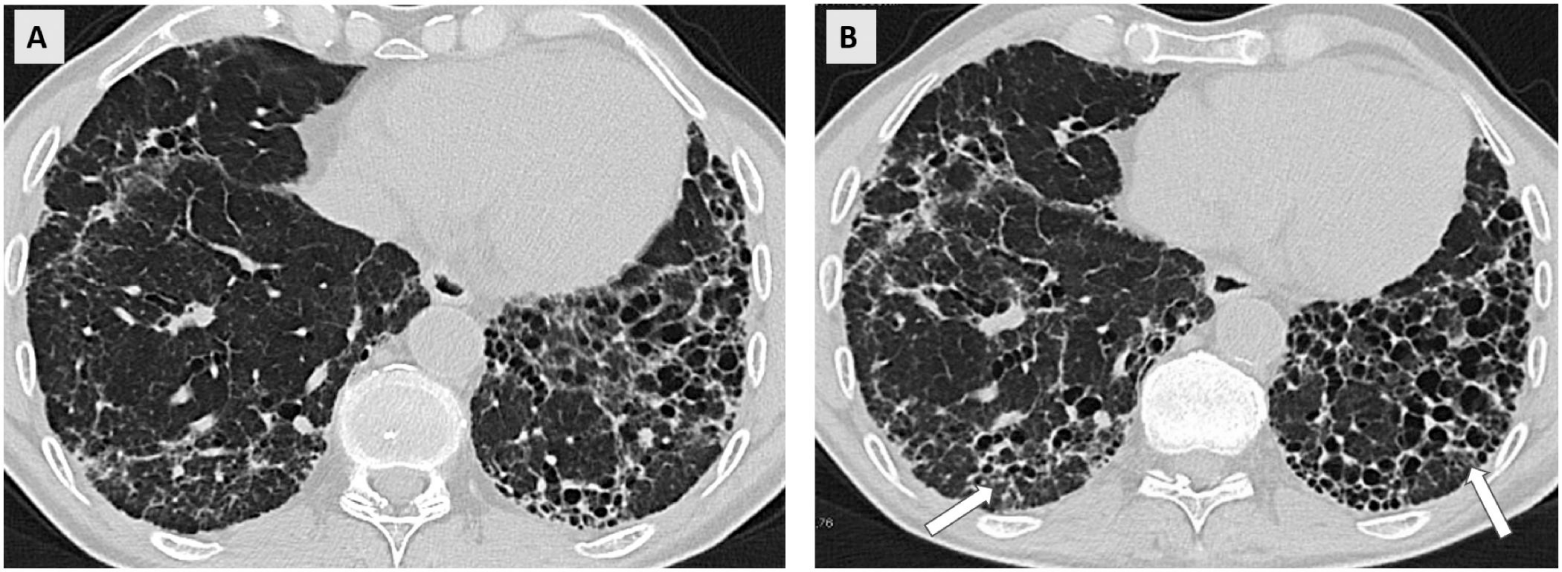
Progressive Pulmonary Fibrosis in a patient with fibrotic hypersensitivity pneumonitis. (A) Axial CT scan of the lower lobes from December 2023. (B) Comparable axial section from a follow-up CT scan obtained in November 2024 due to worsening symptoms showing signs of progressive fibrosis with progressive traction bronchiectasis (arrows) and reticulations.
